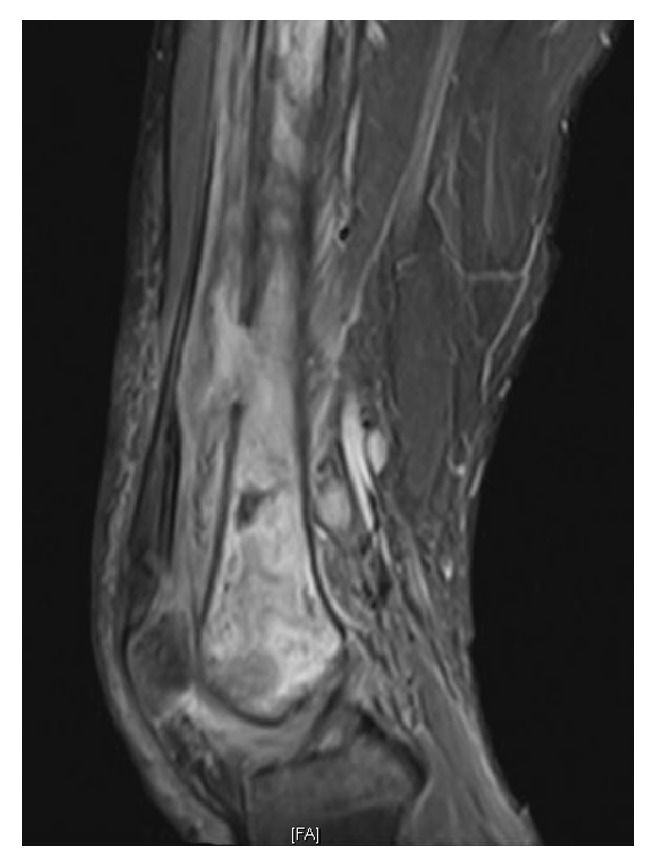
Sagittal MRI of right knee with chronic osteomyelitis from distal femur diaphysis to most distal aspect.
